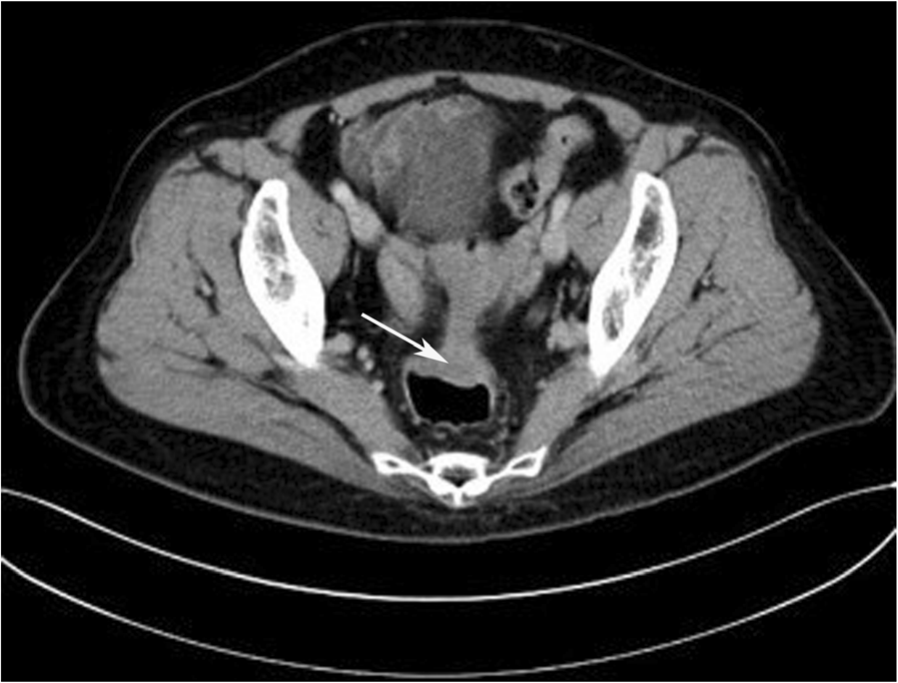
Contrast-enhanced computed tomography (CT) image showing diffuse and irregular thickening of the wall of the recto-sigmoidal junction
